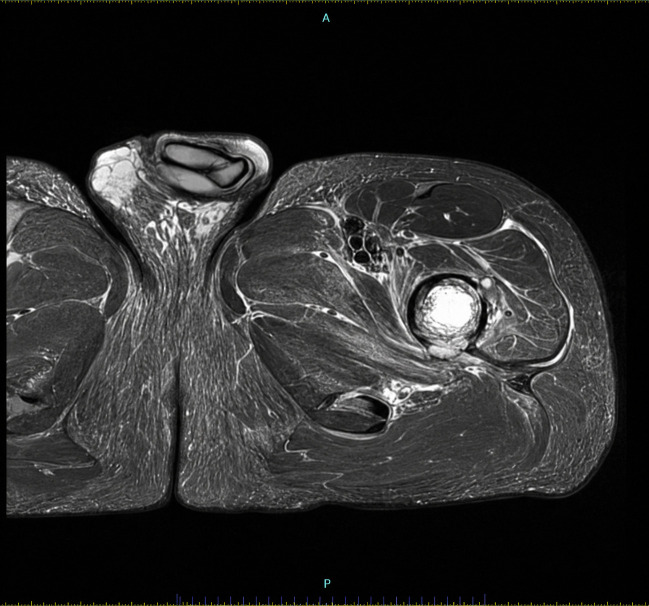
MRI Pelvis transverse (arrow pointing to metastatic lesion in left femur).
